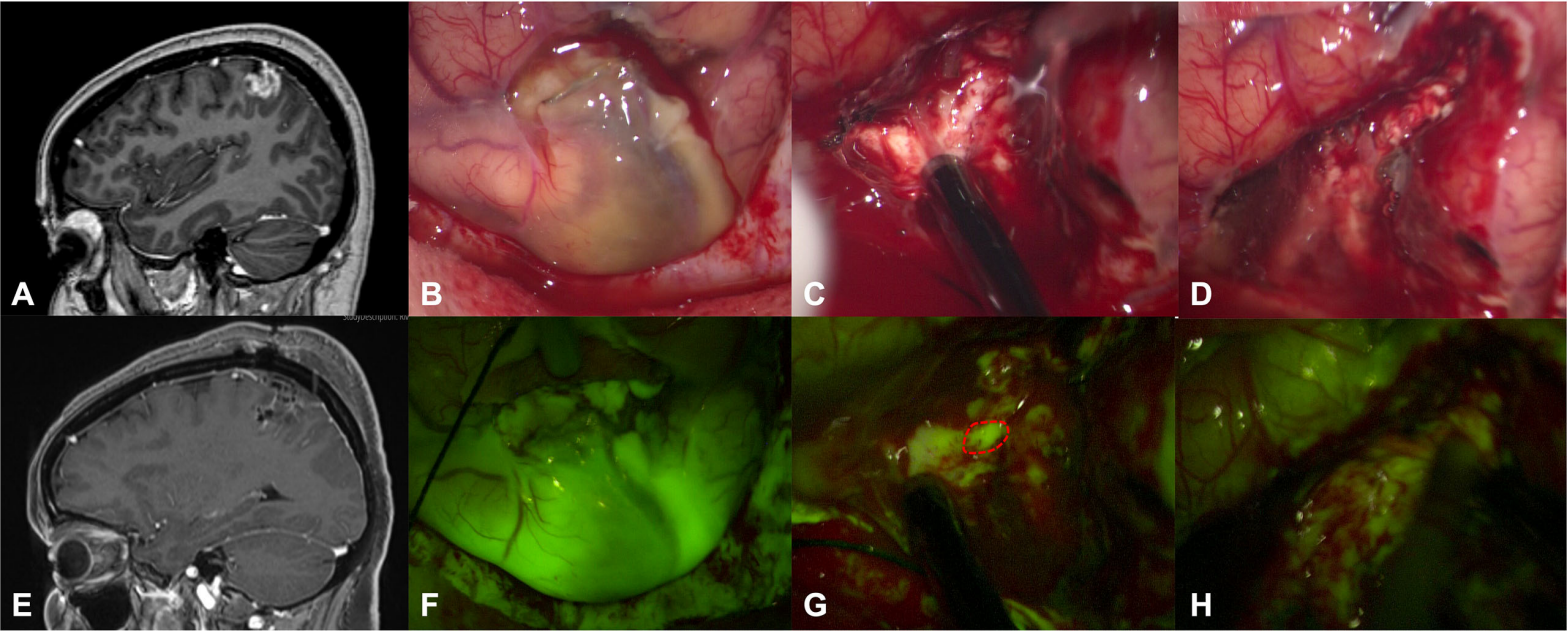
Preoperative T1 with contrast sagittal scan (A) in a 54-year-old female (patient n.8) shows a left frontal anaplastic pleomorphic xanthoastrocytoma with a gross-total resection, as detectable by postoperative post-contrast T1 MRI in (E). After dural opening, it was possible to appreciate a greyish and pale lesion (B) that was better highlighted after activation of YELLOW 560 filter (F). The tumor was removed with an inside-out fashion with the aid of a monopolar suction probe, showing a bleeding, friable tissue (C). During surgical resection, fluorescein helps the surgeon to identify residual pathological tissue (dotted lines in G), not clearly visible under white light (C). At the end of resection (D, H), no more fluorescent/pathological areas were detectable with the YELLOW 560 filter activated or with white light illumination.
